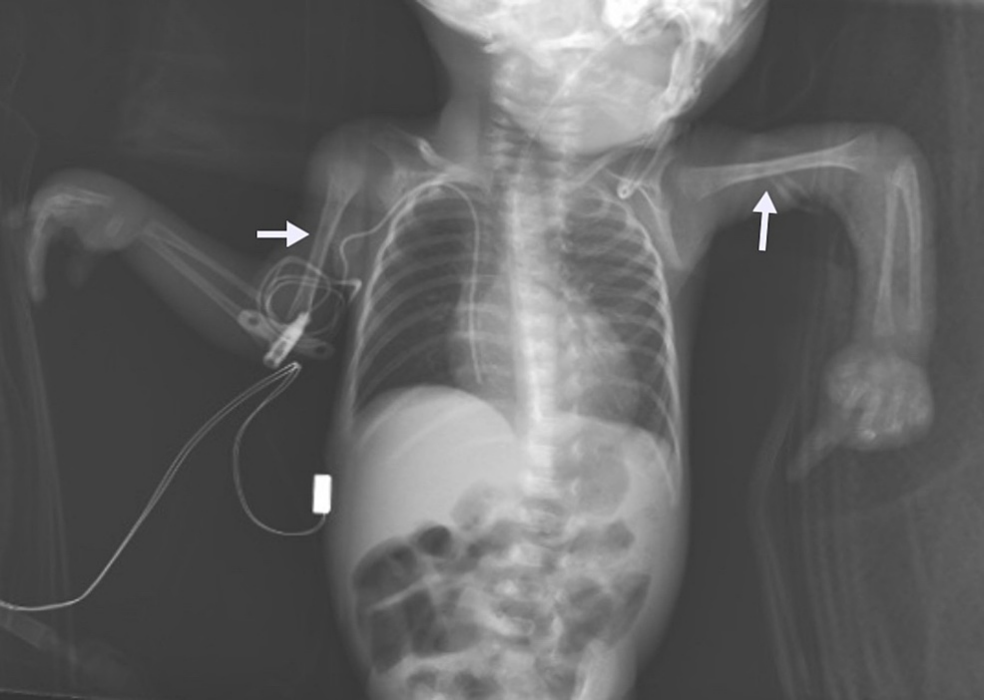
X-ray of the upper body (part of the skeletal survey): anteroposterior view showing shortness of the middle portion of upper limps (mesomelia) (white arrows).
